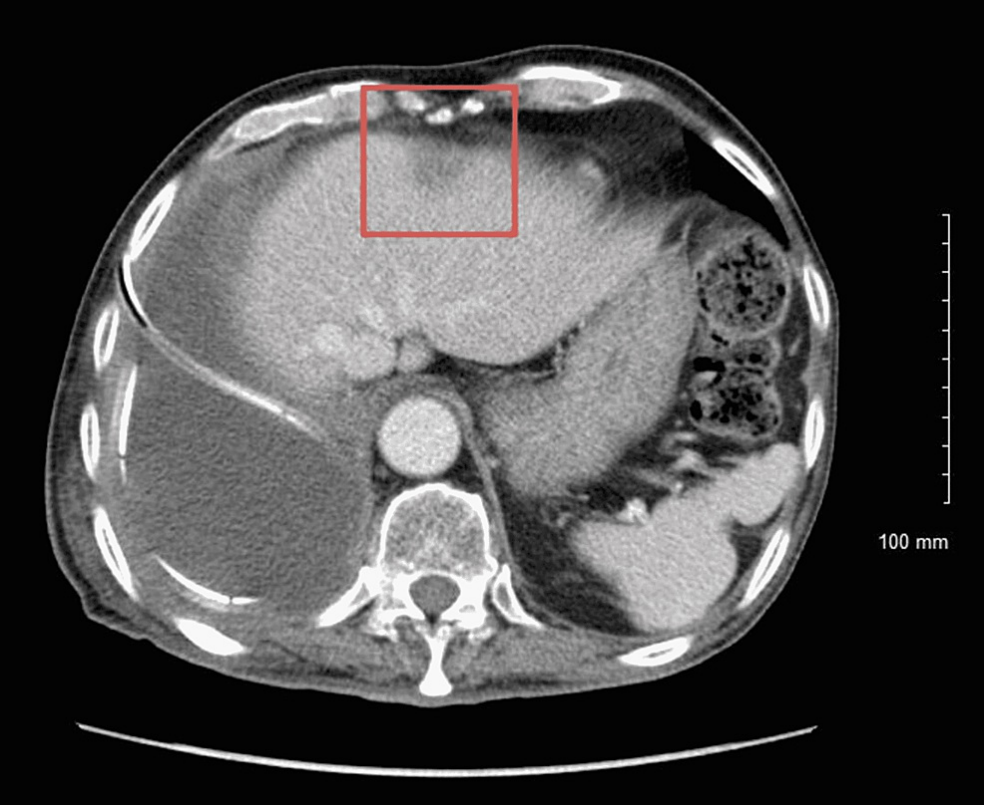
CT scan of the abdomen with contrast showing a 1.6 cm hypodense lesion in the left lobe of the liver.
